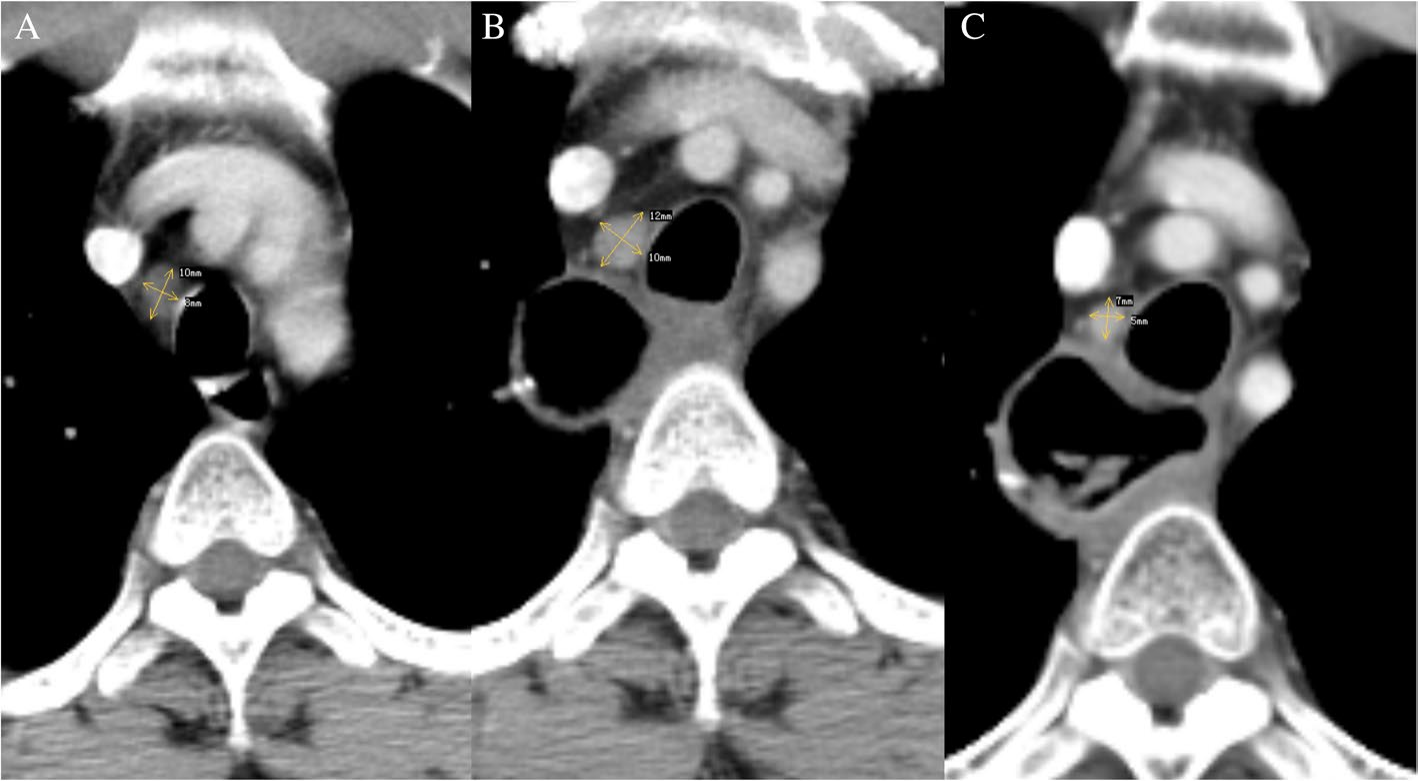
Contrast-enhanced CT image of a 47-year-old man shows a small lymph node in the right upper paratracheal. The short and long diameters are 8 mm and 10 mm in the transverse section on the preoperative CT, with an axial ratio of 0.8 (8/10) (A), this small lymph node was confirmed unresected on postoperative CT 2 months later, with a larger size of 10×12mm in the transverse section (B), and shrunk after radiotherapy with a size of 5×7 mm in the transverse section (C)
